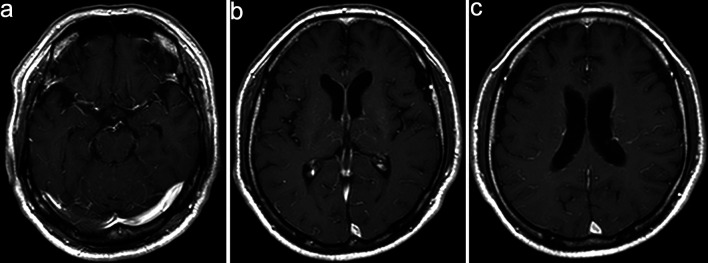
Contrast-enhanced magnetic resonance imaging (MRI) on day 11. (a–c) Contrast-enhanced MRI shows serpiginous perivascular enhancement in the basal ganglia along with leptomeningeal enhancement.
